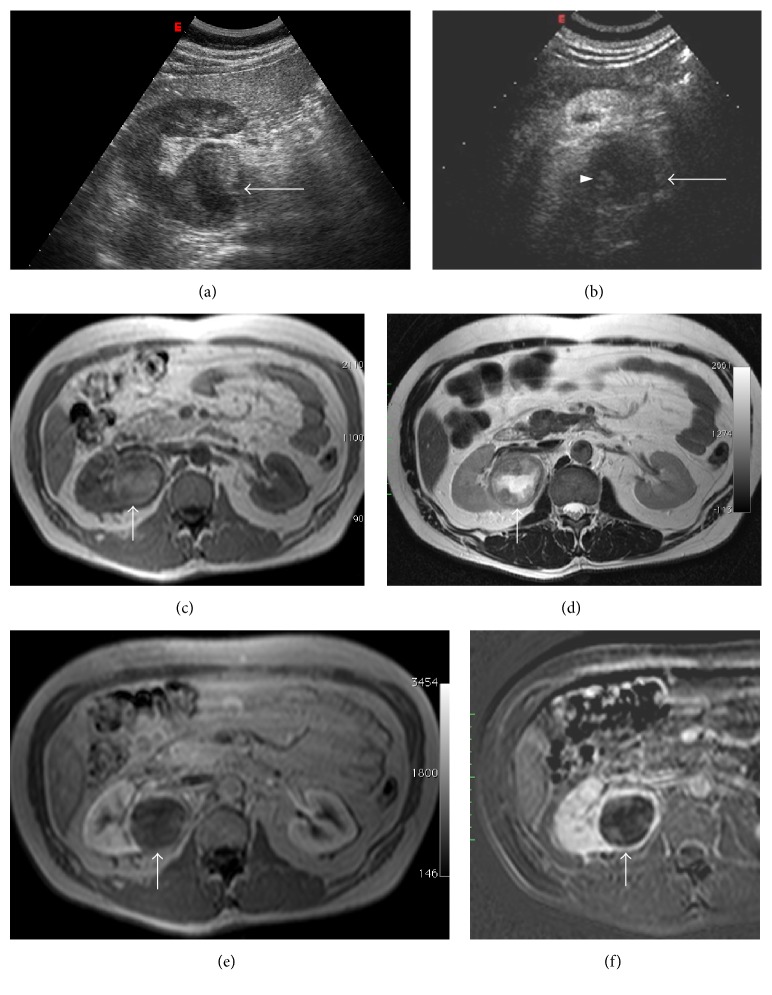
Cystic neoplasm (Bosniak category III). US examination shows a large inhomogeneous hypoechoic mass (arrow) in the third middle of the right kidney (a). At CEUS, enhancement of intralesional septa and nodulations (arrowhead) is also detectable (b). MRI in the same patient (c–f). The mass (arrow) is characterized by an inhomogeneous mild hyperintensity on axial GE T1-weighted image (c) and a central area of hyperintensity on axial T2-weighted TSE image (d). Axial contrast-enhanced GE T1-weighted fat-sat image (e) and subtracted image (f): enhancement of the small solid peripheral component is better depicted on the subtracted image, with similar CEUS appearance.
